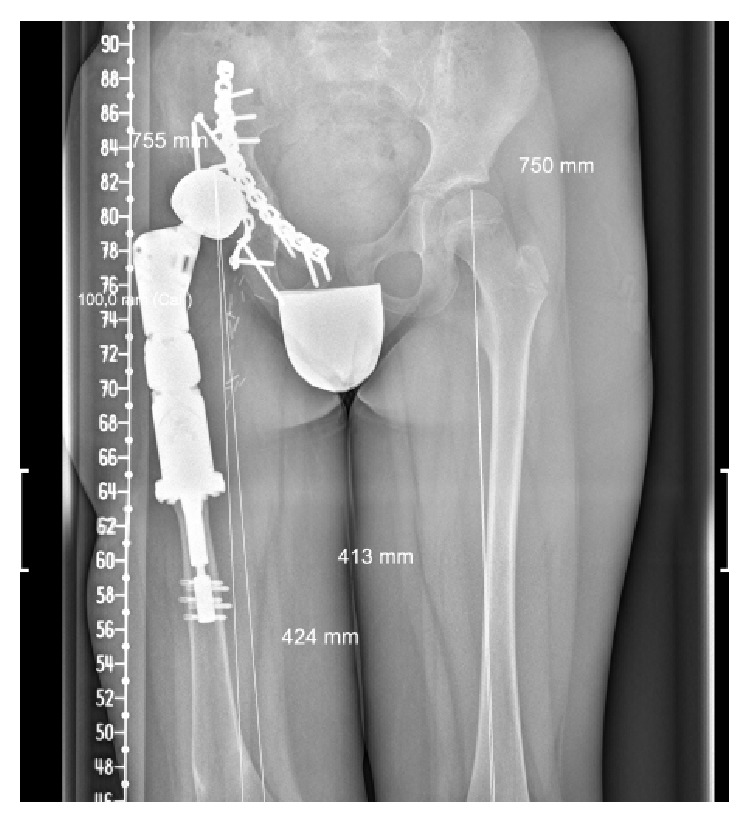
After 18 months of follow-up, a plain AP radiograph of the pelvis shows correct integration of the autograft and prosthesis.
